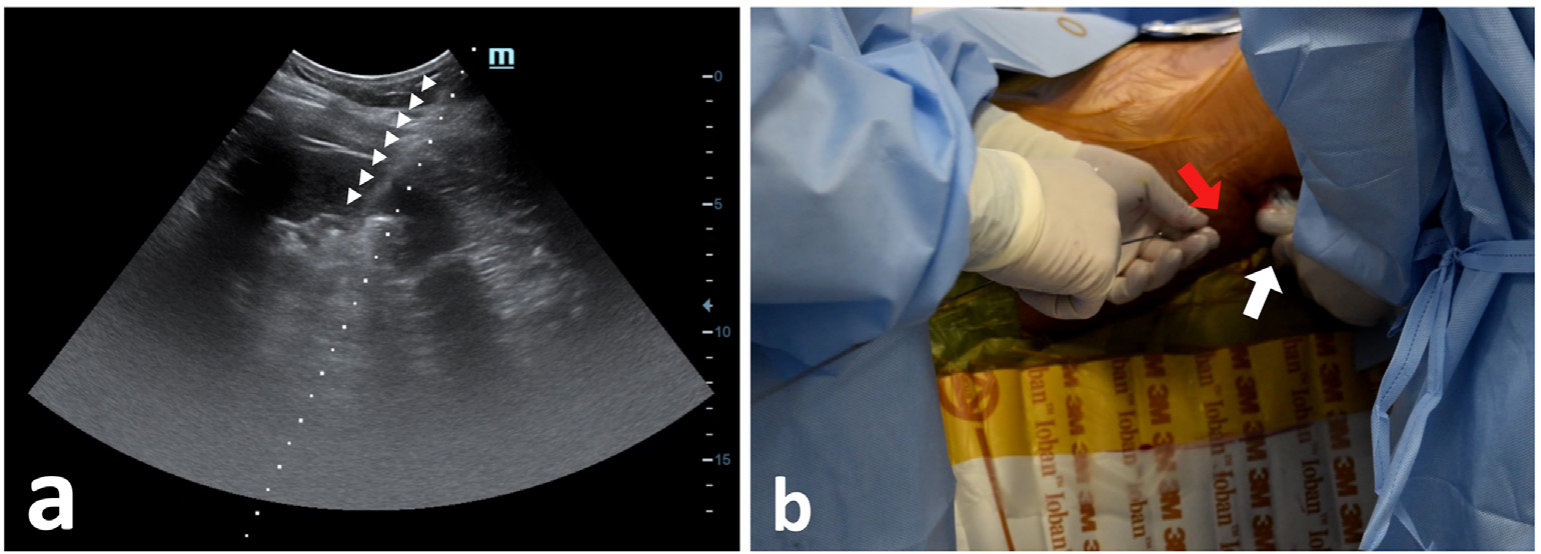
Ultrasound guided renal puncture. (a) Visualisation of Needle tract clearly seen piercing through renal parenchyma, into the pelvic calyceal system. (b) Needle sized 17,5 G, held by operator, puncturing the flank area (red arrow) guided by ultrasound visualization. Ultrasound probe (white arrow) held by the operator assistant.
